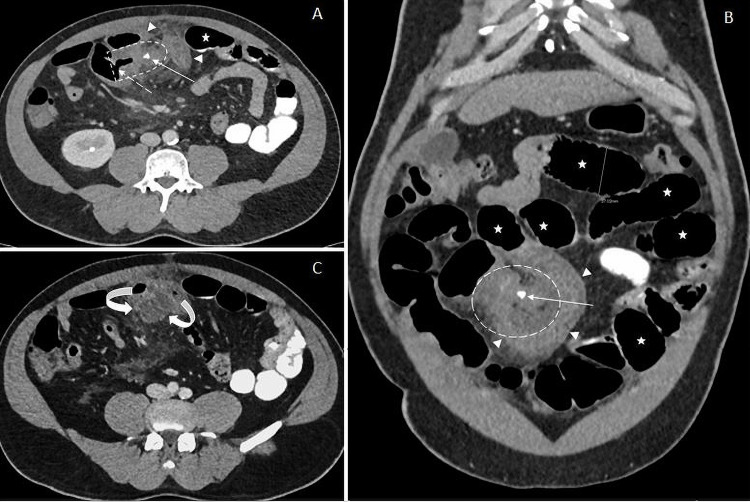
Contrast-enhanced abdominal CT scan. Axial (A) and coronal (B) images show multiple dilated small bowel loops (stars). Short segment of terminal ileum showing narrowed lumen and thickened edematous wall (arrow heads). It forms a U-shaped loop around an ill-defined soft tissue enhancing structure (dashed circle) with central dense focal calcification (arrow) and surrounded by extensive fat stranding and traces of free fluid within the mesentery. This structure is inseparable from the distal part of the ileum and is connected to its lumen through air containing pouch (dashed arrow). Caudal axial section (C) shows small fluid collection (curved arrows) with severe mesenteric fat stranding. The expected shape of the obstructed MD is denoted in image A (dashed delay shape). MD, Meckel's diverticulum
